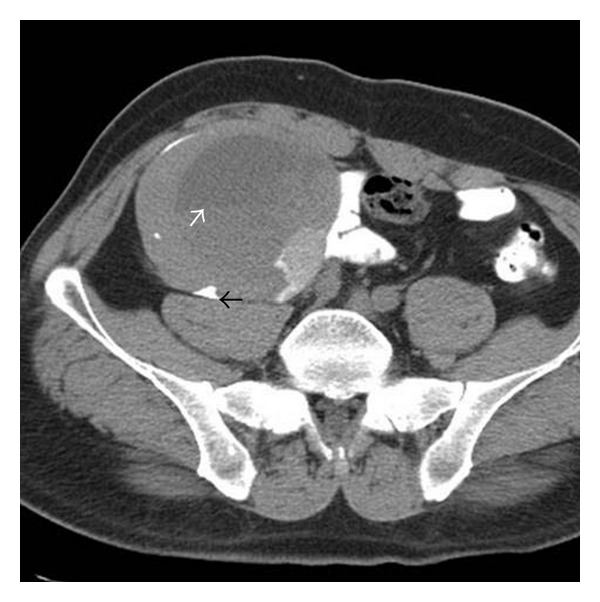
Abdominal precontrast CT shows a thick-walled, cystic, multilocular mass, measuring 10 × 10 cm in front of the right psoas and iliopsoas muscles and adjacent to the iliac vessels. Spotty calcification (black arrow) was seen in the thick, hyperdense wall. White arrow shows fluid-fluid levels.
